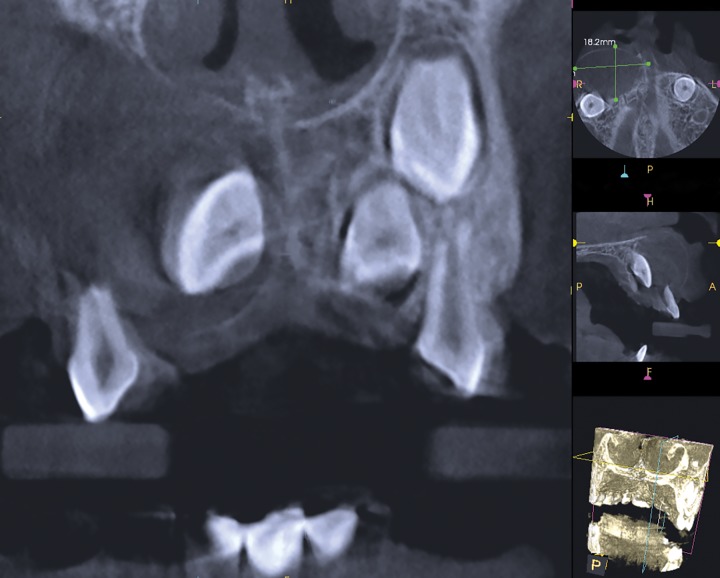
Cone beam computed tomography showing the radicular cyst causing thinning of the nasal floor
